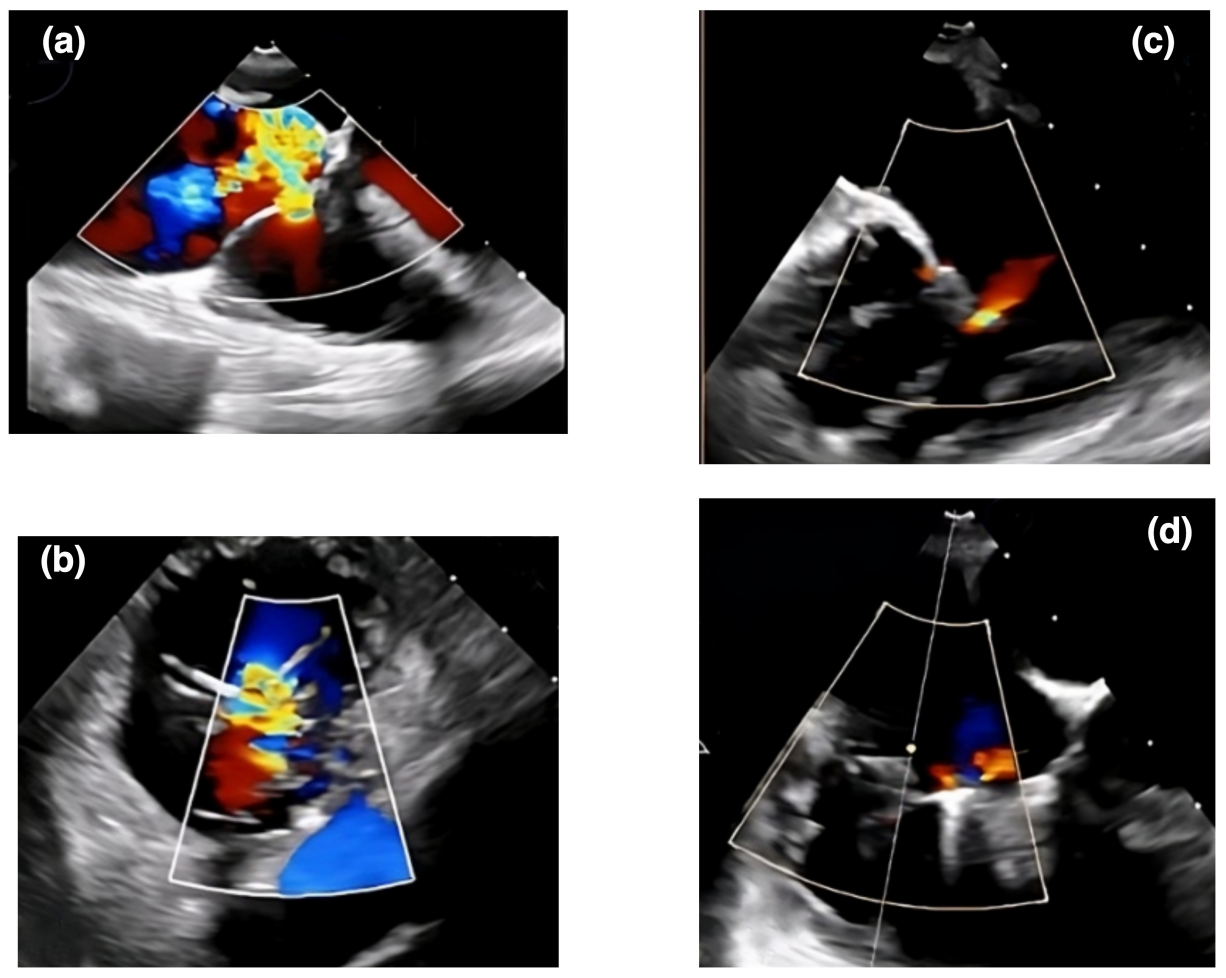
An echocardiographic representation of a transcatheter
